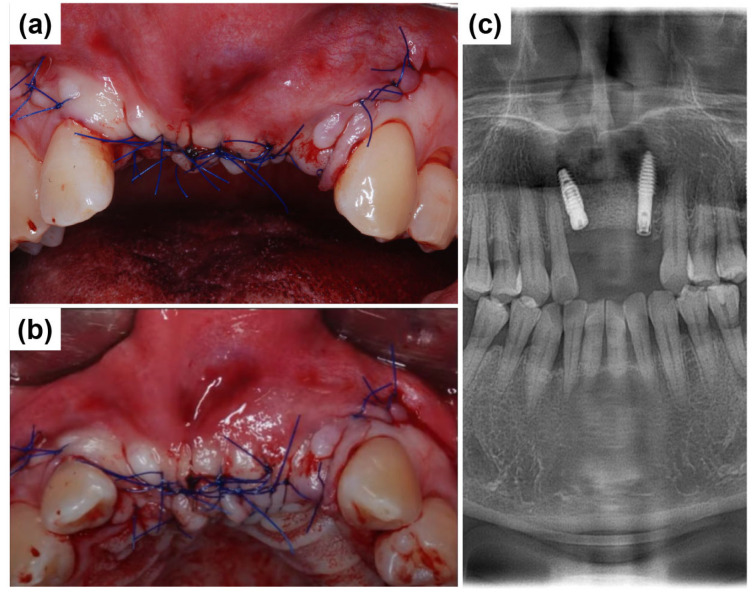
Primary closure and postoperative radiographic assessment. (a) Labial intraoral view showing flap repositioning and primary closure using 4-0 Vicryl sutures following implant placement. (b) Occlusal intraoral view. (c) Postoperative panoramic radiograph depicting the final placement of implants in the anterior maxillary region.
